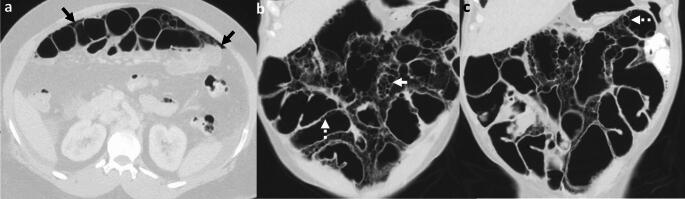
Pneumatosis cystoides intestinalis. A 57-year-old with systemic sclerosis, mild abdominal pain, and flu-like symptoms. CT images show pneumoperitoneum (black arrows, a) multiple submucosal cysts in the colon (solid white arrow, b) and small bowel (dotted white arrows, b, c). There was a distinct absence of peritoneal signs, and the patient was managed conservatively
